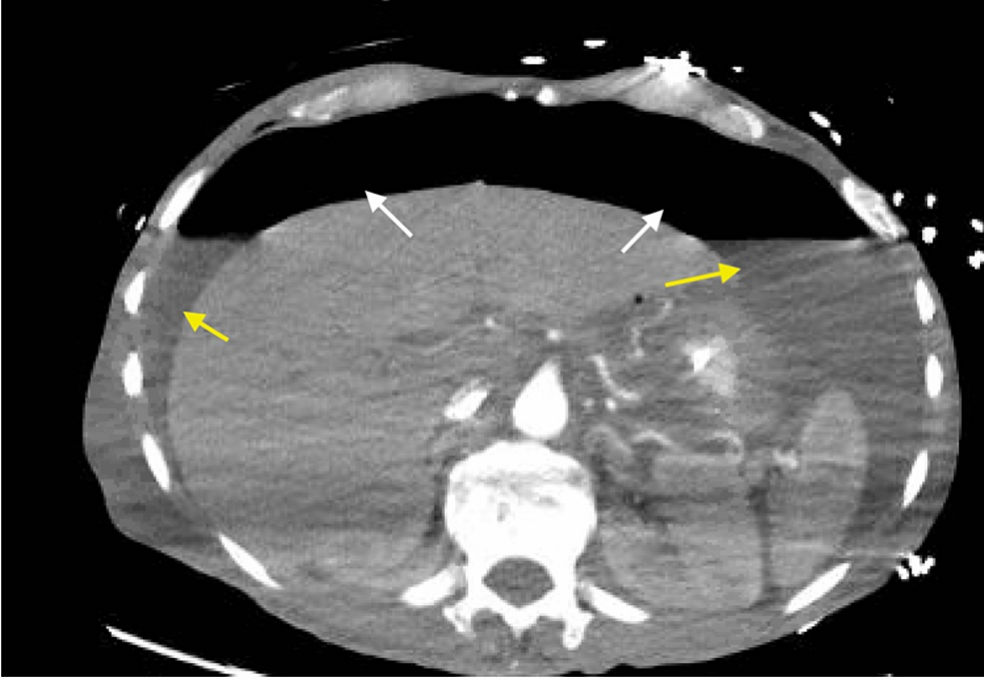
CT of the abdomen with IV contrast showing free intraperitoneal air (white arrows) and ascites (yellow arrows).
